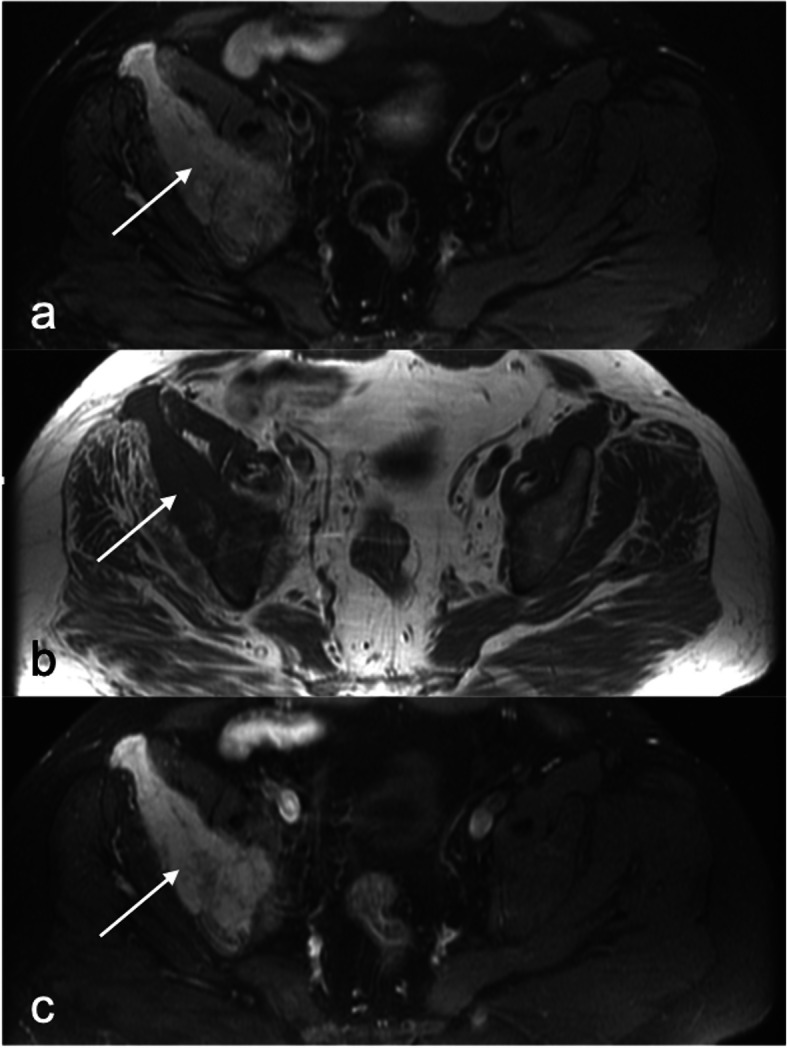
Magnetic resonance imaging of the pelvis showing the expansile and destructive lesion affecting the right iliac bone (arrows), hyperintense on proton density Fat Sat weighted image (a), of intermediate signal with some normal bony streaks on T1 weighted image (b) and showing extensive contrast enhancement after IV injection of gadolinium (c)
